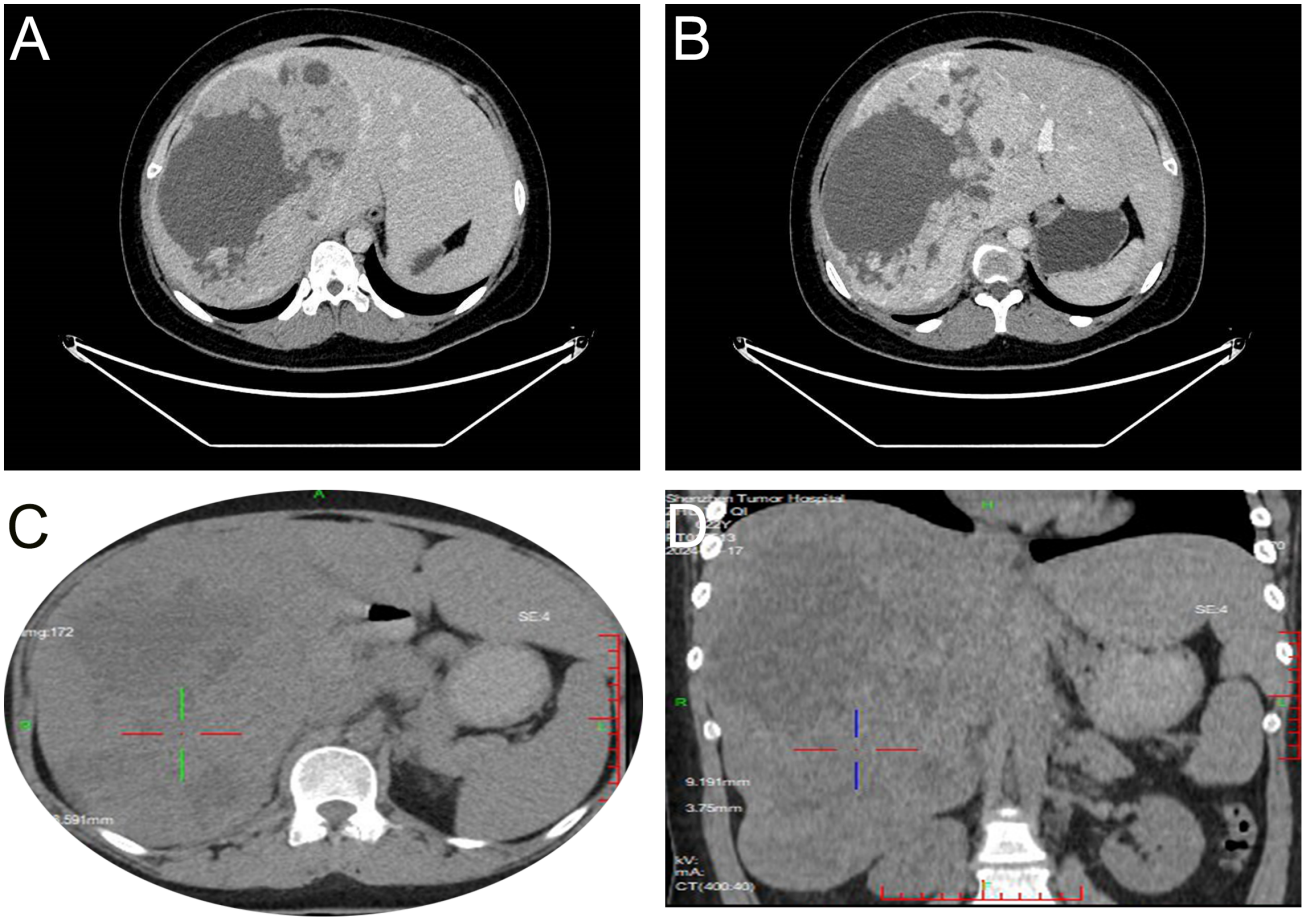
Imaging results. CT shows the tumor tissue located in the right lobe of the liver, with clear boundaries and mixed slightly low-density appearance with multiple patchy low-density shadows and a small amount of calcification (A). After enhancement, the arterial phase shows unevenly pronounced enhancement (B). PET-CT reveals a mixed-density mass in the right lobe of the liver, partially fused into a cluster, with unevenly increased uptake (1C, D).
